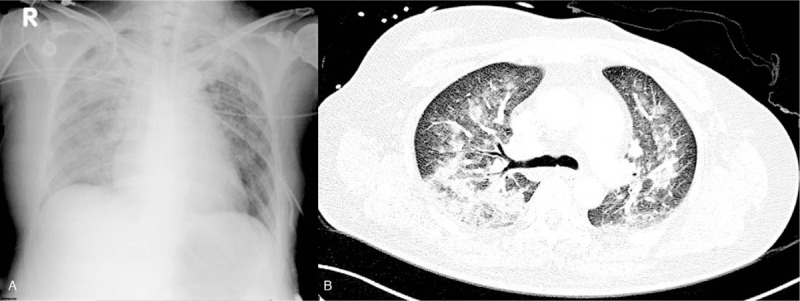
(A) Postoperative plain chest roentgenograms reveal bilateral lung consolidation. (B) Chest computed tomography image (lung window) of the patient with severe diffuse alveolar hemorrhage shows symmetrical bilateral peribronchial alveolar pattern with interspersed ground-glass opacities indicative of pulmonary edema.
